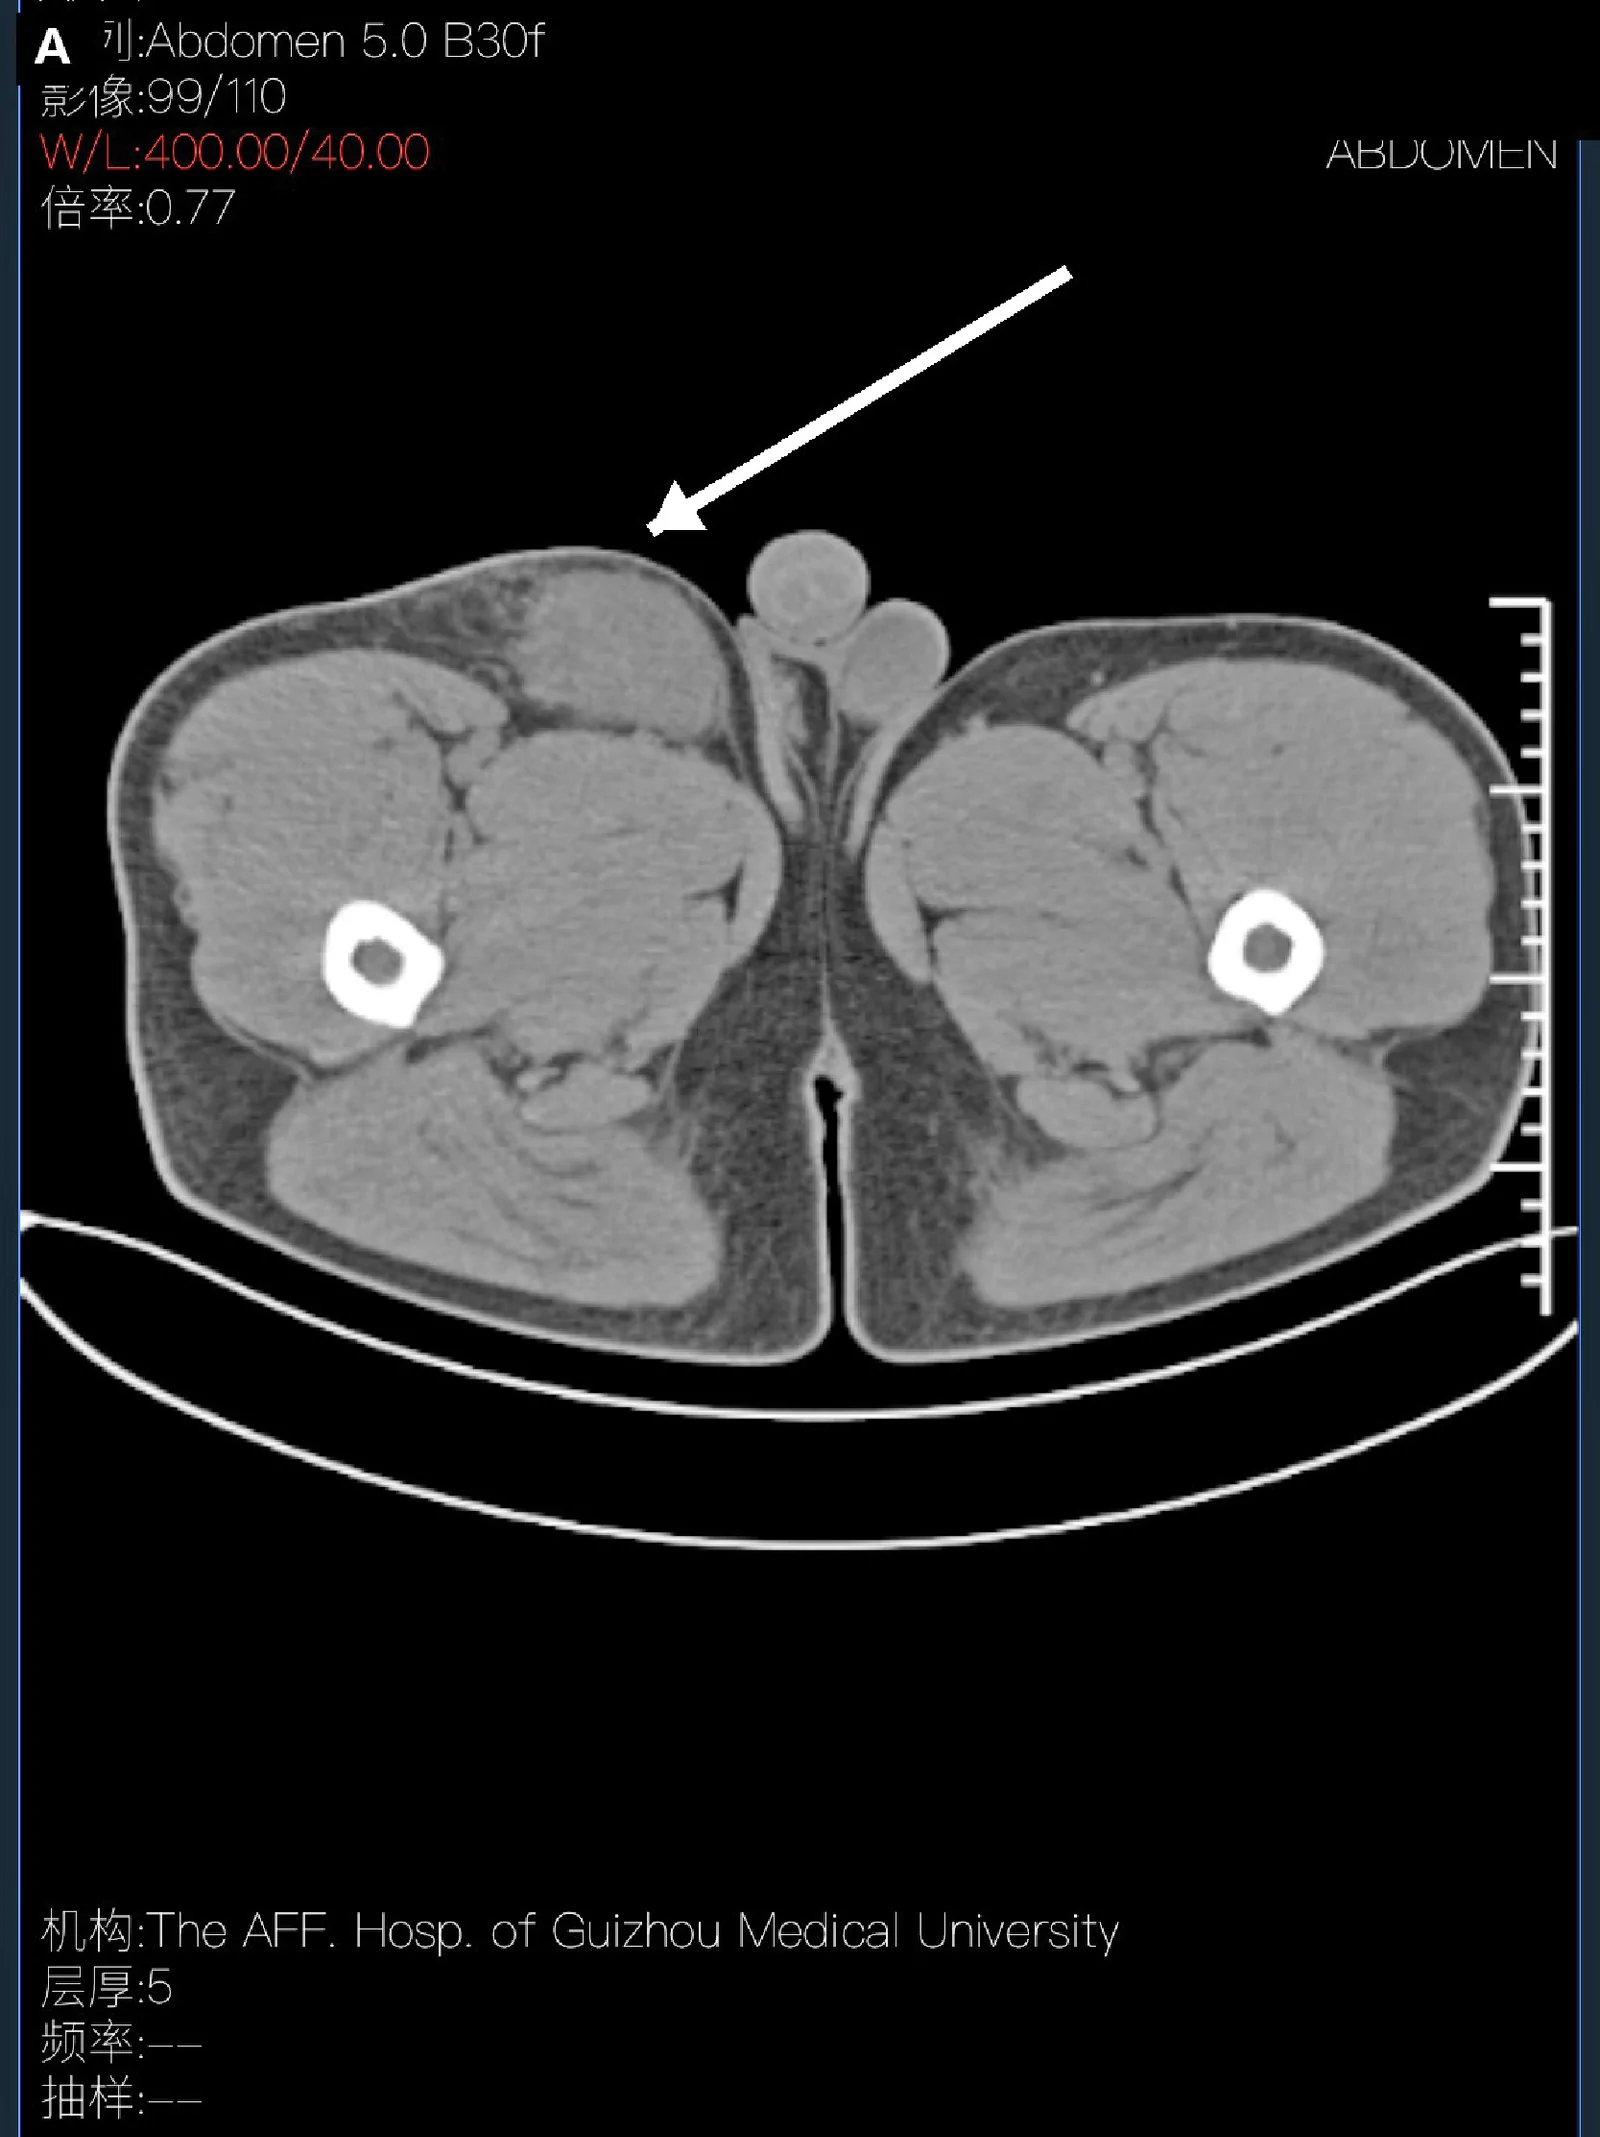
Axial noncontrast CT image of the inguinal region. The white arrow indicates the dominant right inguinal subcutaneous soft-tissue mass. The sagittal image from the original submission was removed because the lesion was incompletely included and poorly centered.
